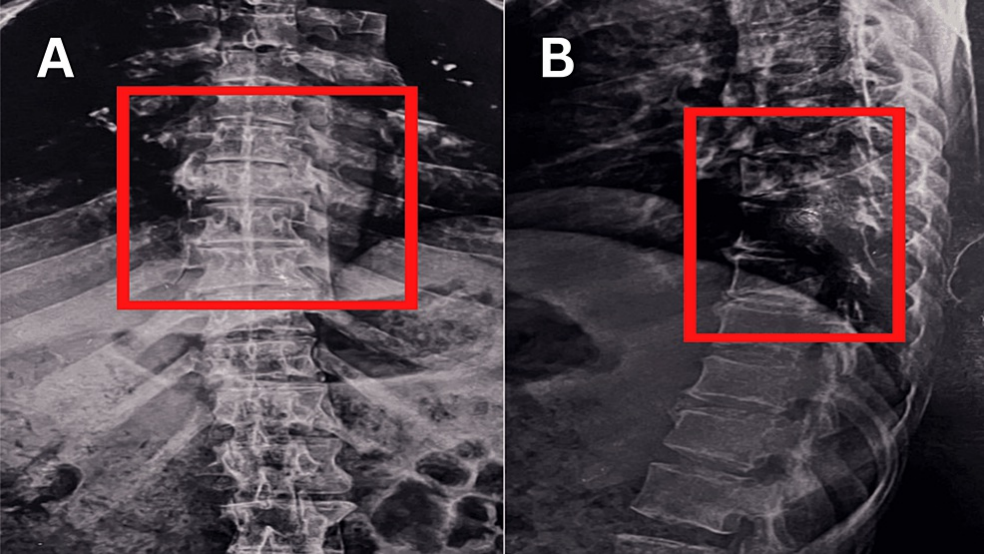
The one-year-old spine X-ray image The image is showing a D7 wedge compression fracture. (A) Postero-anterior view (B) Lateral view D7: Seventh dorsal vertebrae
